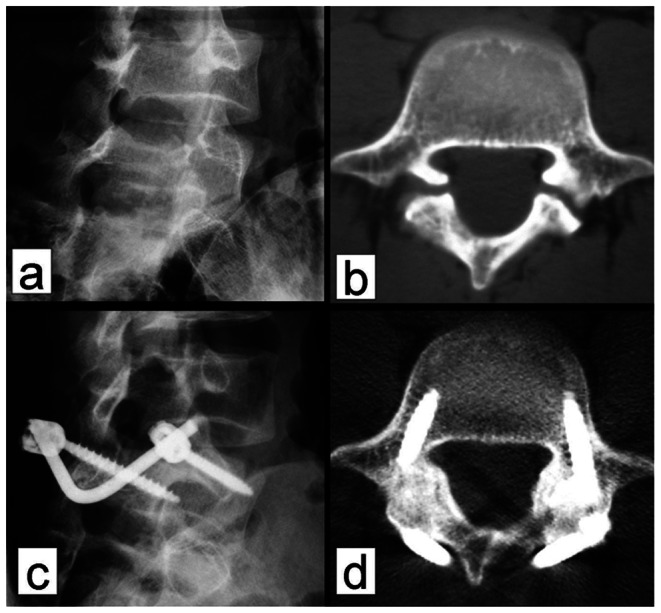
A 22-year old male patient with isthmic spondylolysis for 2 years failed conservative therapy. Preoperative oblique x-ray and CT scan using bone windows reveal isthmic spondylolysis at L5 (a and b). At 6 months postoperatively, the oblique view shows an indistinct interface between the isthmus and bone graft with increased density (c). CT scan on bone windows shows healing of the isthmic bone graft (d).
